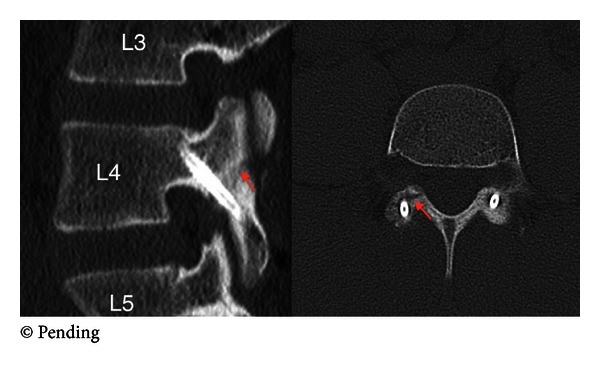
Three-month post-operative CT shows appropriate intraosseous screw placement with healing of the pars defect and no signs of nonunion.
